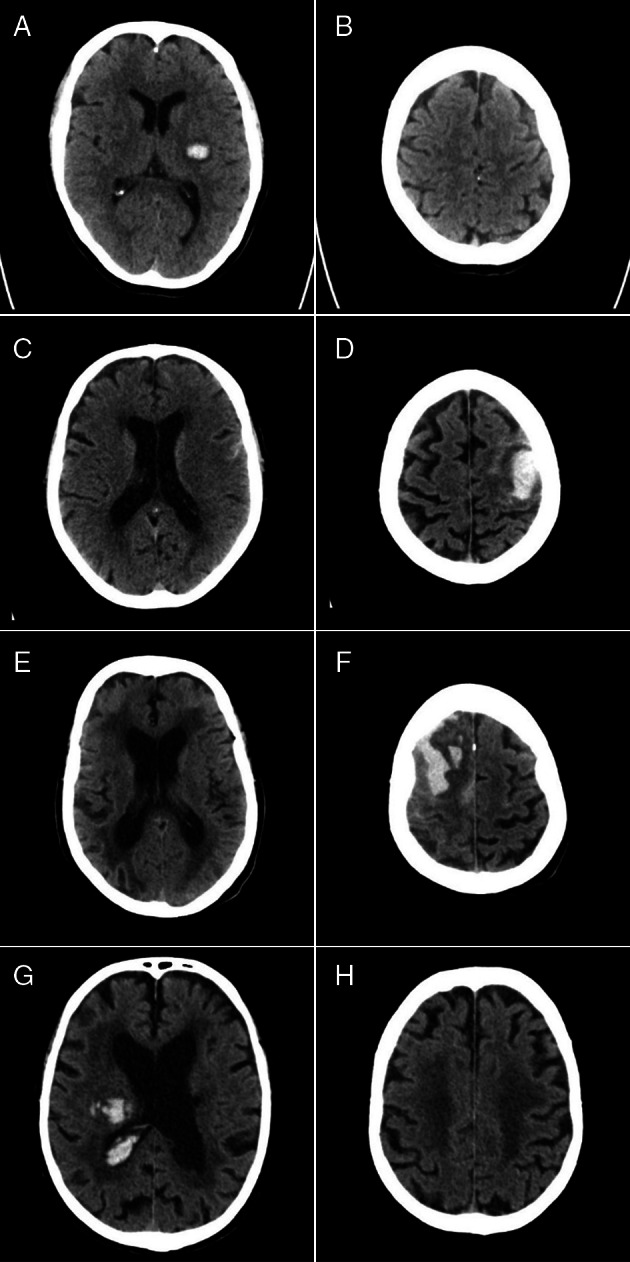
Example axial noncontrast brain computed tomographic (CT) images at the level of the lateral ventricular choroid plexus (A, C, E, and G) and centrum semiovale (B, D, F, and H) showing the range of CT small vessel disease (SVD) scores in patients with first‐ever SVD–intracerebral hemorrhage (ICH). (A, B) CT SVD score 0 (anterior white matter lucencies [WMLs] = 0, posterior WMLs = 0, no lacunes, central atrophy = 0, cortical atrophy = 0). (C, D) CT SVD 1 (anterior WMLs = 1, posterior WMLs = 1, no lacunes, central atrophy = 1, cortical atrophy = 2). (E, F) CT SVD 2 (anterior WMLs = 2, posterior WMLs = 2, no lacunes, central atrophy = 2, cortical atrophy = 2). (G, H) CT SVD 3 (anterior WMLs = 2, posterior WMLs = 2, 4 lacunes [one of which is visible in the left corona radiata], central atrophy = 2, cortical atrophy = 1).
